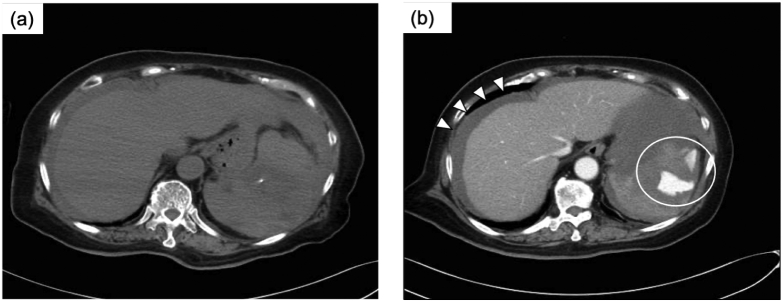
CT of the abdomen on admission Simple CT (a) and contrasted CT (b) of the abdomen showed hematogenous ascites effusion (arrowhead) not only around the liver and spleen but also in the pelvis and extravasation from the cranial side of the spleen implicating active bleeding (white circle). CT, computed tomography.
